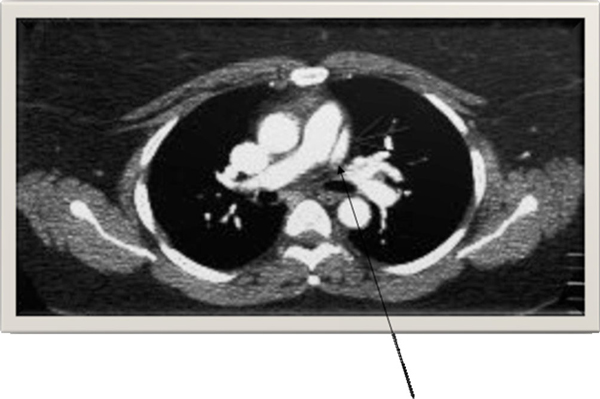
Computed tomography of the chest with contrast showing a linear hypodensity within the main pulmonary artery suggestive of an intimal dissection flap of the trunk of the main pulmonary artery without dilation.
